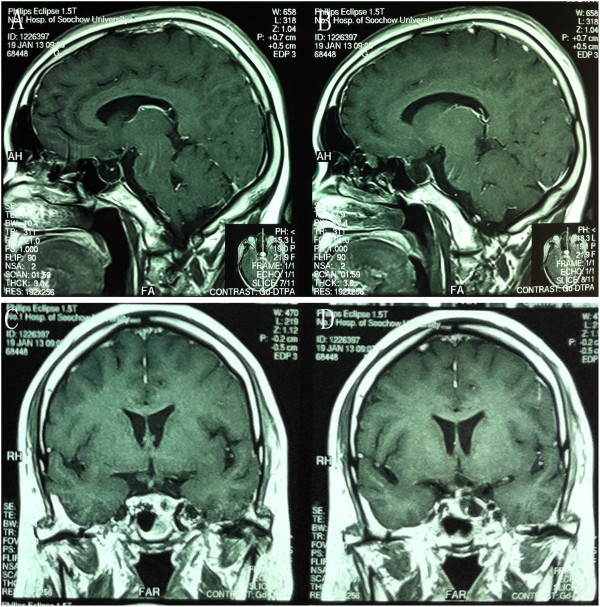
Case number 10: Axial (A-B) and coronal (C-D) view of contrasted magnetic resonance imaging showing total removal of pituitary adenoma.
